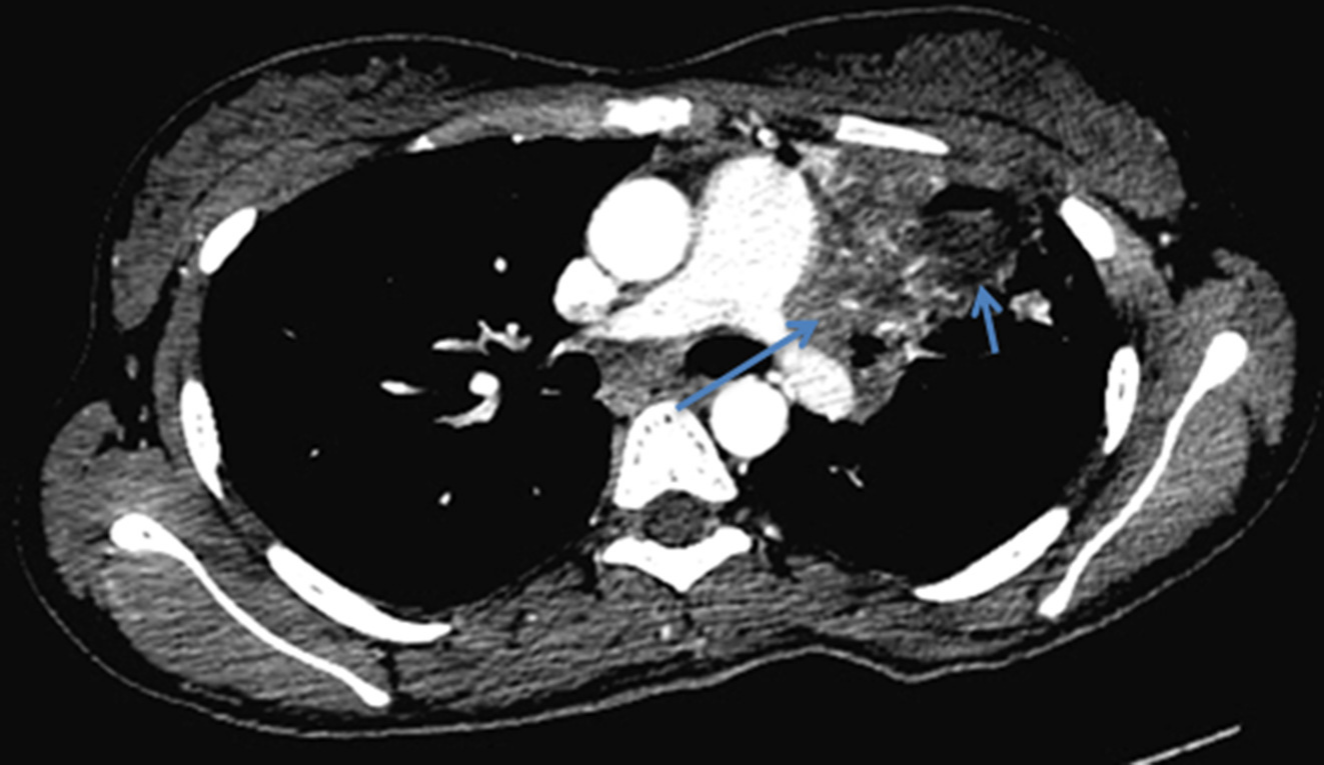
Case 2(d): Axial post-contrast CT image of the chest in mediastinal window shows cavitating consolidation (short arrow) in the left upper lobe with extension into the hilum and narrowing of the hilar structures (long arrow).
